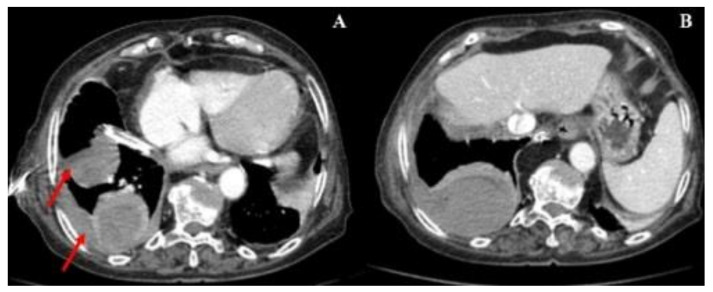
Thoracic-abdominal-pelvic CT showing two lymphomatous masses in right superior lobe with pleural origin (red arrows); no splenomegaly or intra-abdominal and mediastinal lymphadenopathies (A); Thoracic-abdominal-pelvic CT showing right pleural effusion, after thoracentesis and intravenous diuretic therapy (B).
